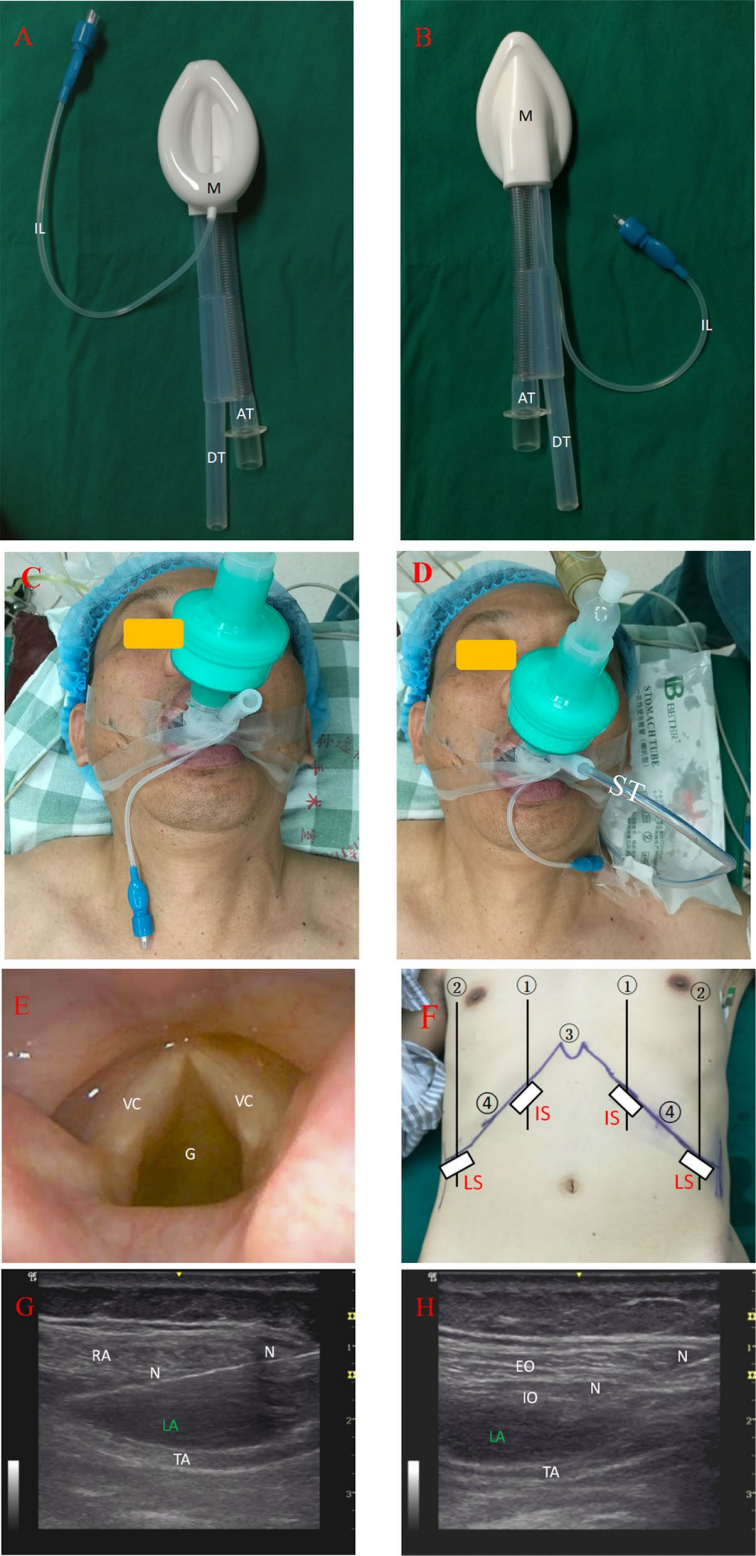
Procedures of LMA ventilation and subcostal TAP block. (A) Double-tube LMA: ventral side; (B) Double-tube LMA: back side; (C) Placement of LMA; (D) LMA ventilation and esophageal drainage; (E) LMA positioning by fiberoptic bronchoscopy; (F) positions of ultrasound probe for TAP block; (G) Ultrasound image of TAP block: inner site; (H). Ultrasound image of TAP block: lateral site. *AT* airway tube, *DT* esophageal drainage tube, *M* mask, *IL* inflation line, *ST* stomach tube, *G* glottal, *VC* vocal cord, ①: parasternal line, ②: anterior axillary line, ③: xiphoid, ④: costal arch, white rectangle: ultrasound probe, *IS* inner site, *LS* lateral site, *N* needle, *LA* local anesthetic, *RA* rectus abdominis, *TA* transversus abdominis, *EO* obliquus externus abdominis, *IO* obliquus internus abdominis.
